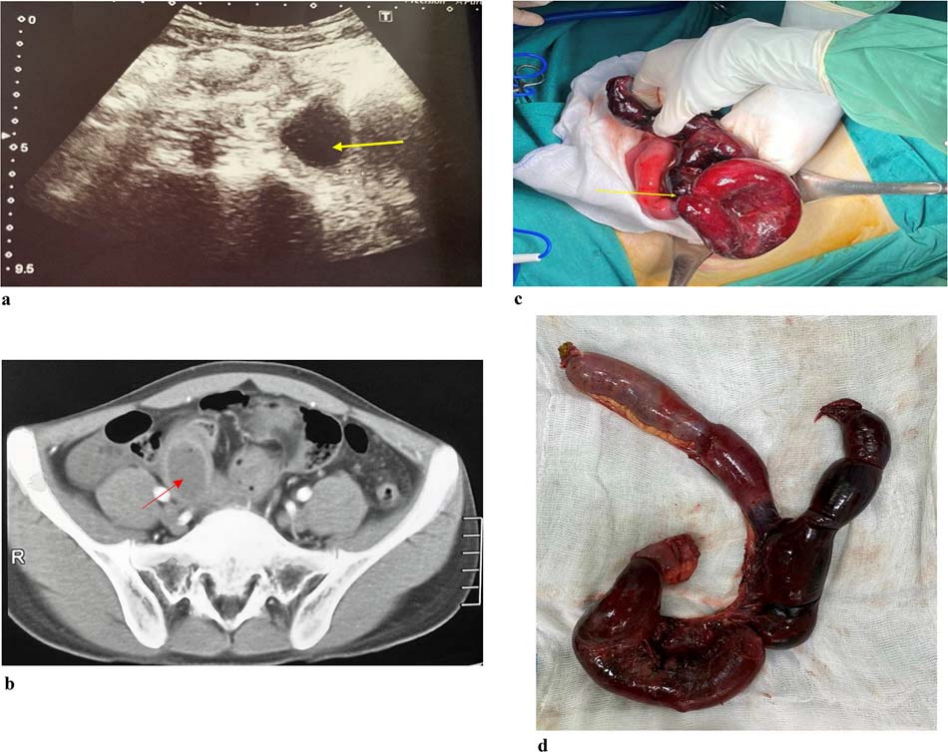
(a). Ultrasound shows an arrow pointing to anechoic structure with hypoechoic wall. (b) Axial CT scan showing an arrow pointing to an ileal cyst dilation with bowel ischaemia. (c) Intraoperative image of small bowel ischaemia with an arrow pointing to the strangulated location. (d) Postoperative image shows ileal duplication cyst.
